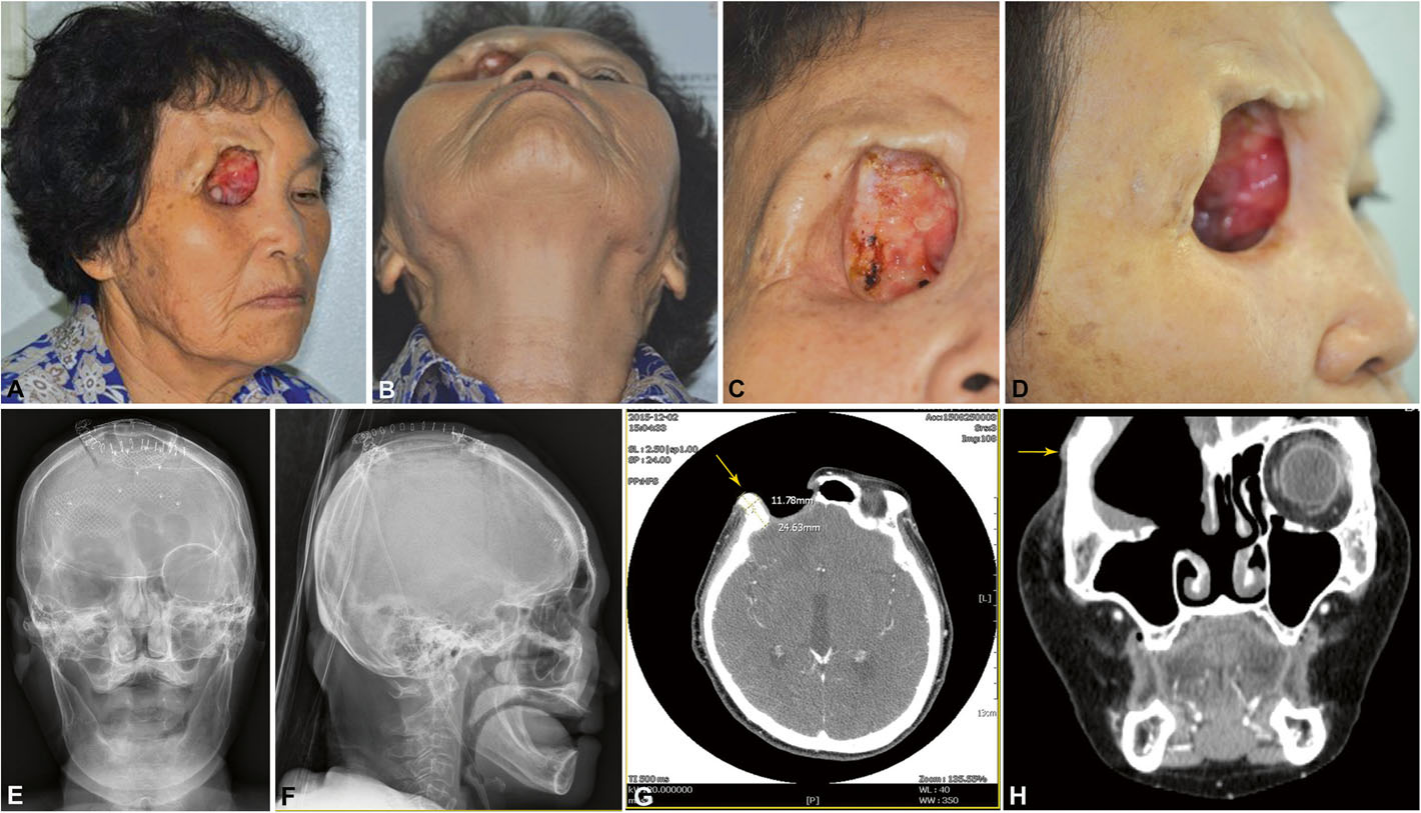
The patient’s clinical and radiographic views after orbital exenteration. The orbital defect on the right side of the face (a–d), frontal (e), and lateral (f) skull views, and computed tomography axial (g) and coronal (h) views are shown along with measurements of residual bony dimensions
